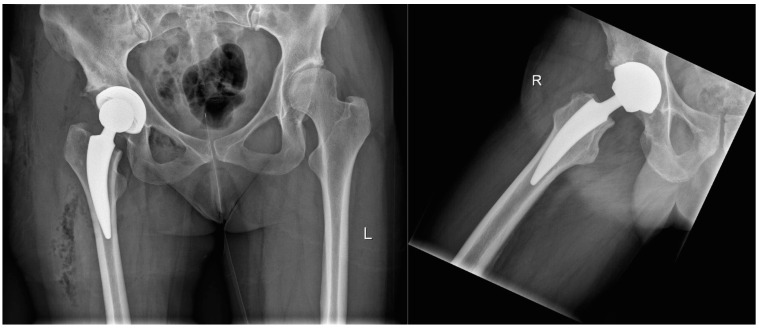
Immediate postoperative situation after MiniMIS implantation on the right site, radiograph a.p. and axial.
